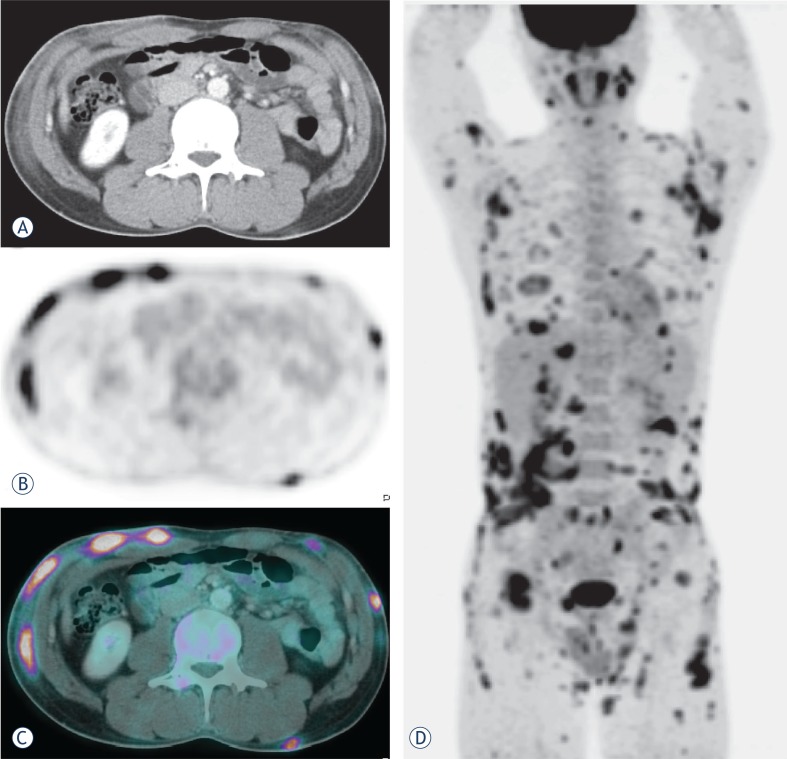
Axial contrast-enhanced CT (A), PET (B), and fusion PET/CT (C) images performed for initial staging demonstrated areas of abnormal increased F-18 FDG uptake corresponding to infiltrative changes in the subcutaneous adipose tissue of the anterior abdominal wall. Extensive disease involvement throughout the body with numerous subcutaneous nodules is best visualized on the maximum intensity projection (MIP) image (D).
